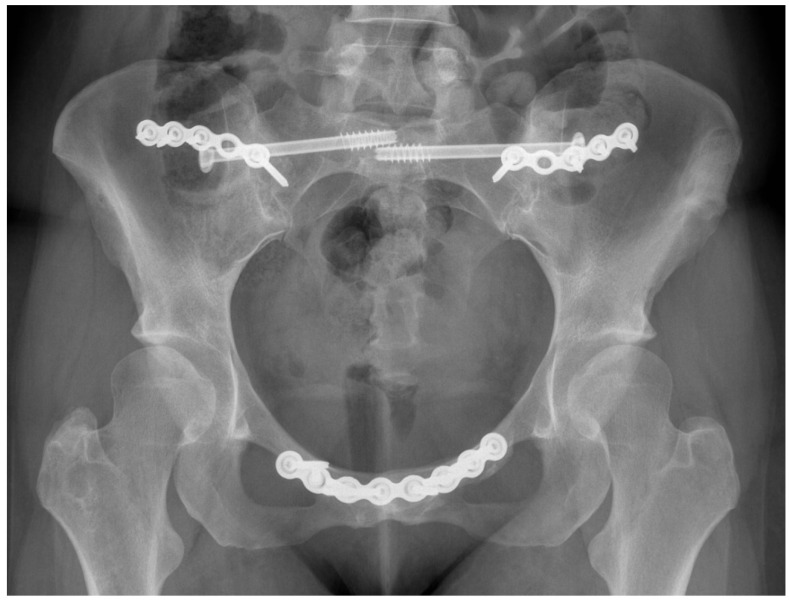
Anteroposterior pelvic radiograph of a 30-year-old female diagnosed with Ehlers-Danlos Syndrome demonstrating fusion of pubic symphysis and both sacroiliac joints (anterior plating, bone grafting and sacroiliac screw insertion).
